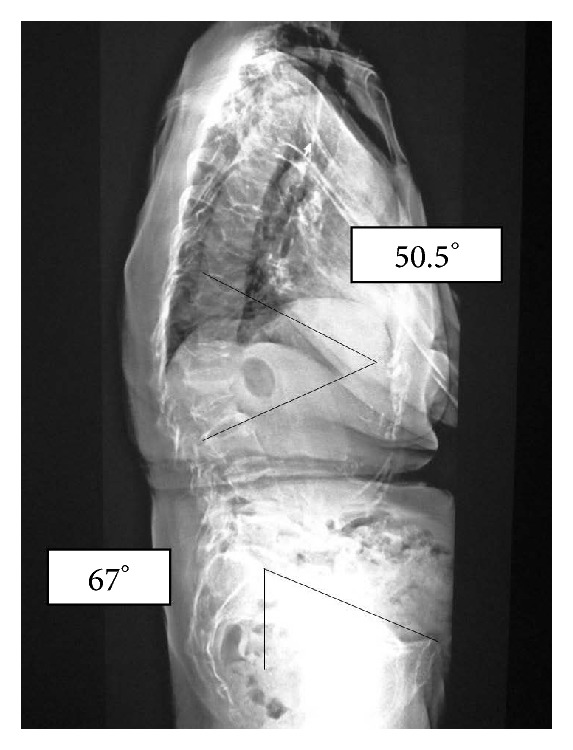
Slot radiography of the spine in the sagittal plane in a standing position. The T10-L2 thoracolumbar angle is 50.5°. Sagittal pelvic tilt angle is 67°. This image reveals severe posterior pelvic tilt following thoracolumbar kyphosis due to vertebral fractures.
